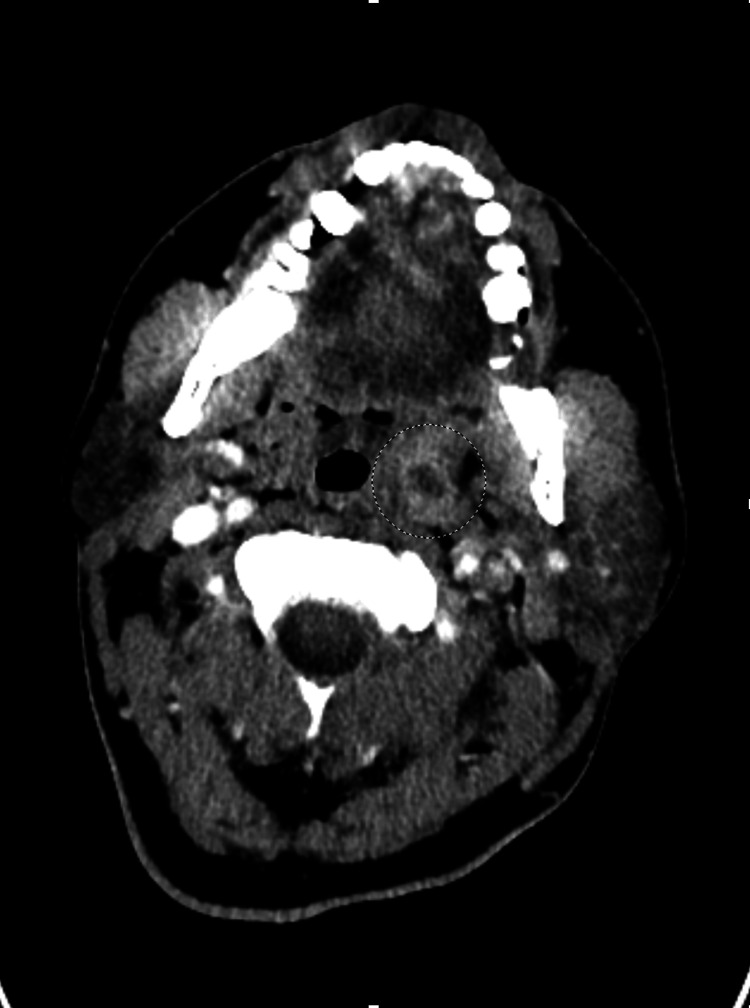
Repeat axial contrast-enhanced CT imaging showing the formation of a 1x1 cm peritonsillar abscess.
